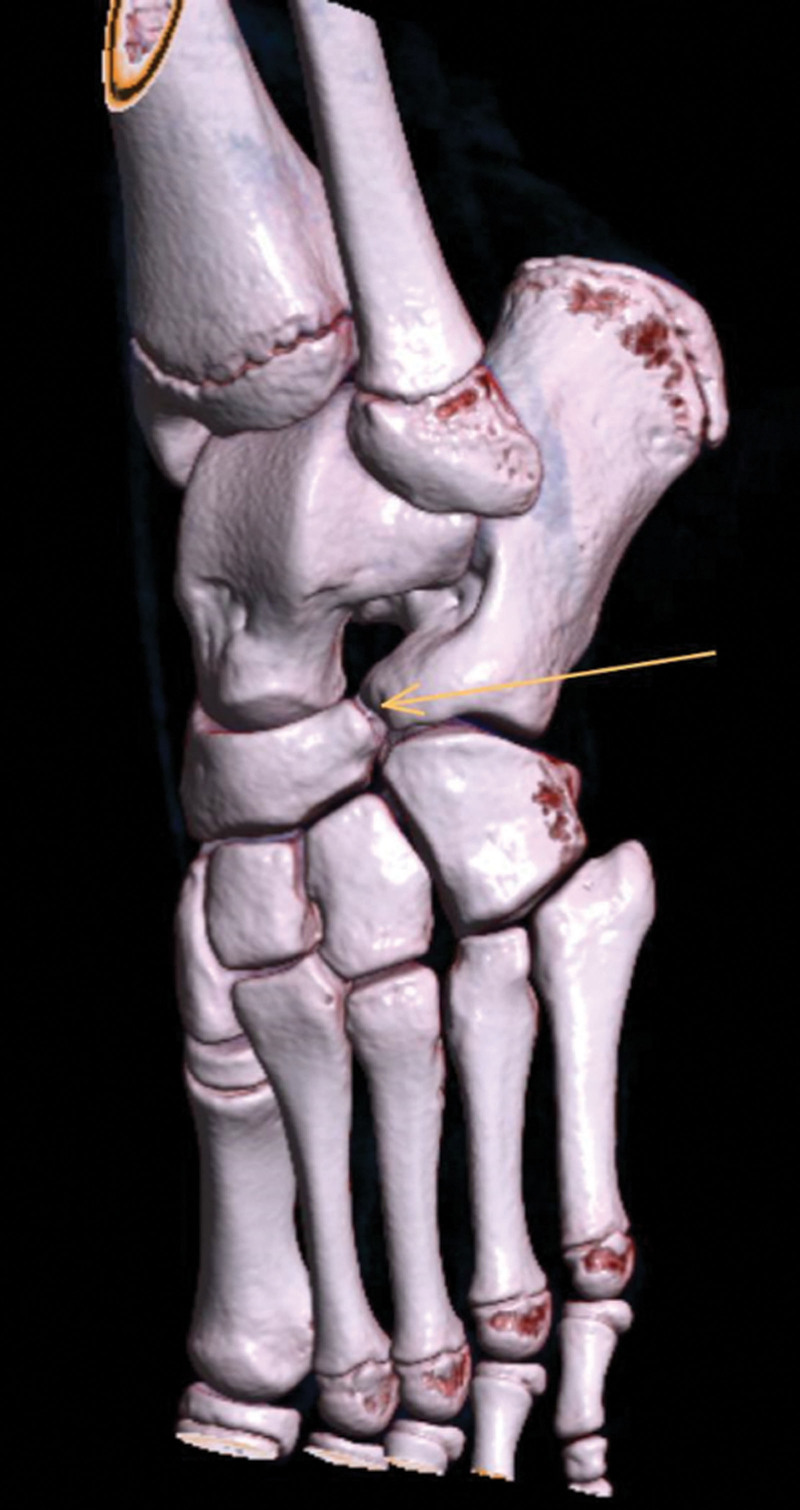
3D CT image of a calcaneonavicular coalition.
